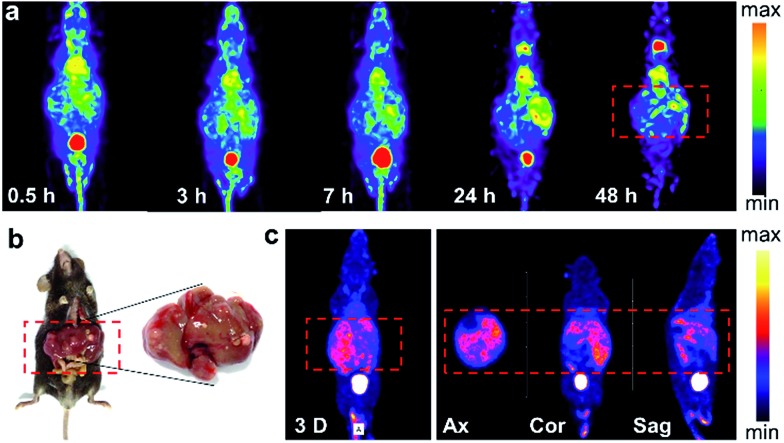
(a) SPECT images of ^125^I–Pd nanosheets in the *Mst1/2* double-knockout hepatoma model. (b) Photographs of liver tissue. (c) SPECT images of the *Mst1/2* DKO tumor model after i.v. injection with the ^99m^Tc-GSA probe.
